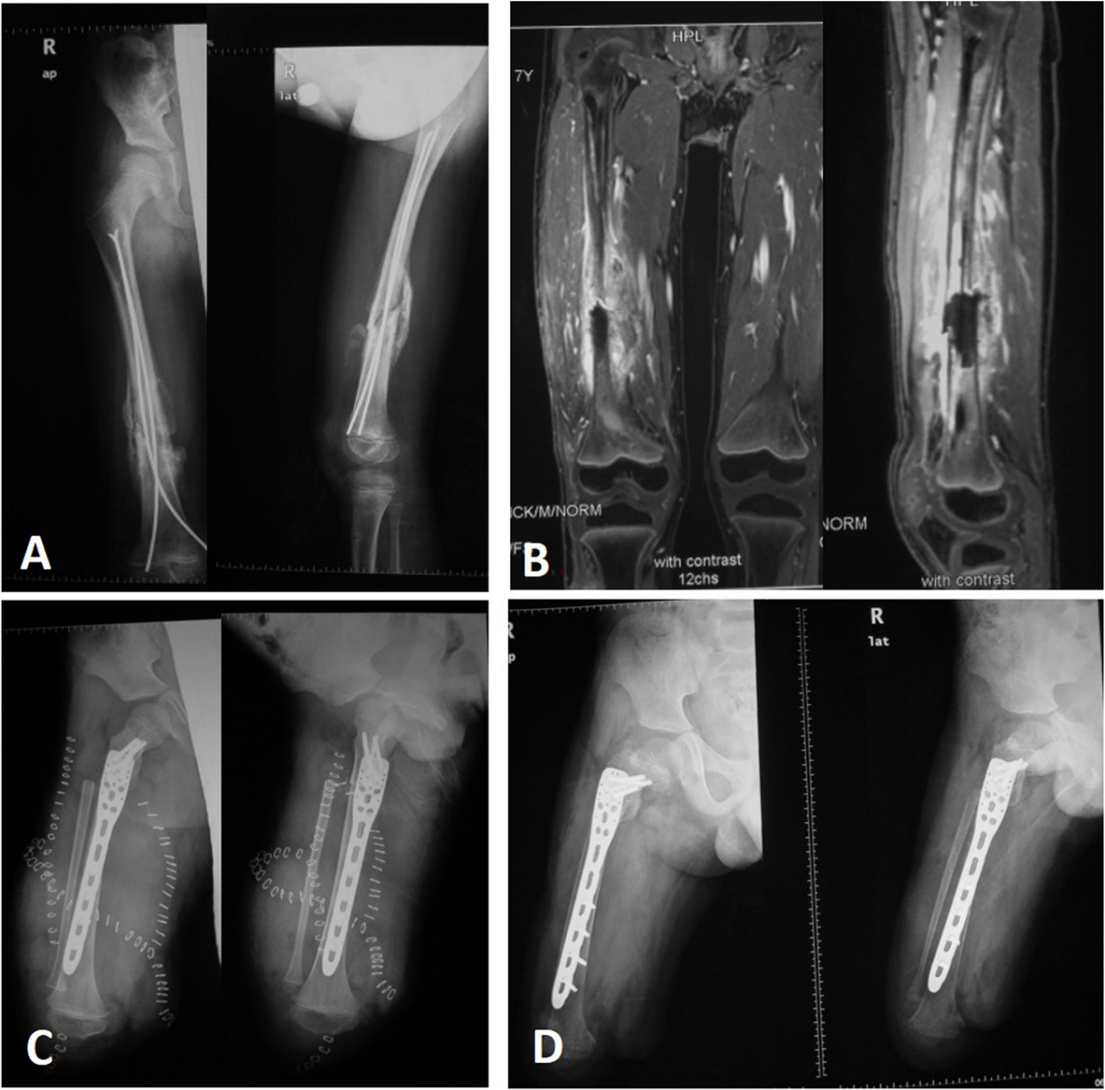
Plastic lengthening amputation of the proximal femur in a 7-year-old boy. a The contaminated operation was performed in the femur of a patient with osteosarcoma who was misdiagnosed with traumatic fracture and intramedullary fixation with two Steinmann pins. b Coronal and sagittal MRI views show that the contaminated pins reached just below the greater trochanter (5.5 cm stump left). c X-ray shows that the tibia was fixed to the remnant femur with an addition of 18 cm to the stump after the operation. d X-ray was performed 2 years after the operation
